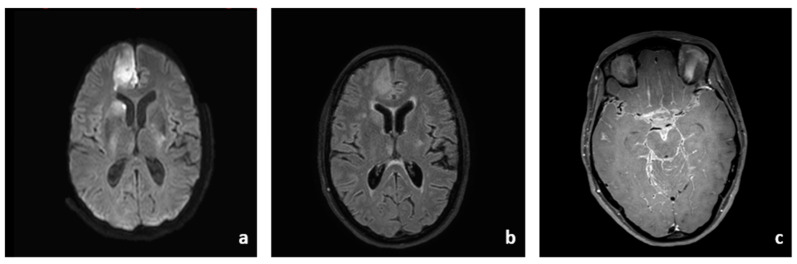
MRI findings at admission. Cerebral MRI shows subacute ischemia in the territory of the right ACA and MCA ((a): DWI, (b): FLAIR). Vessel wall enhancement of the ICA, MCA and ACA indicates vasculitis ((c): black blood + gd).
